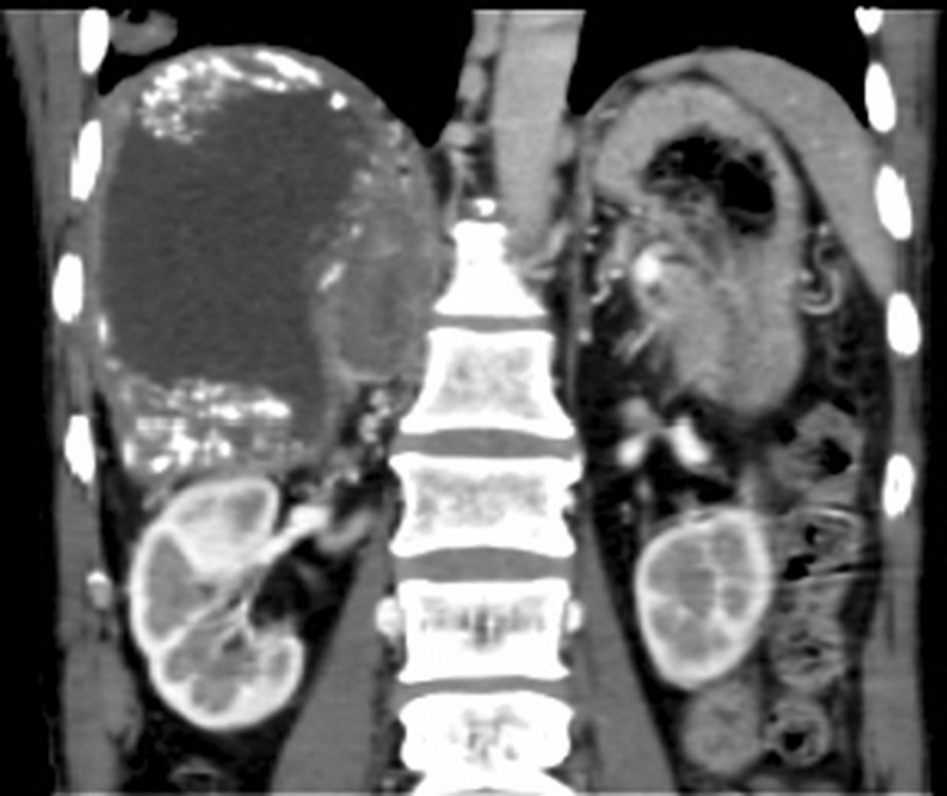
Hepatic AE involved the right adrenal gland. A mixed density mass in the right adrenal gland was visible in the coronal CT image.
